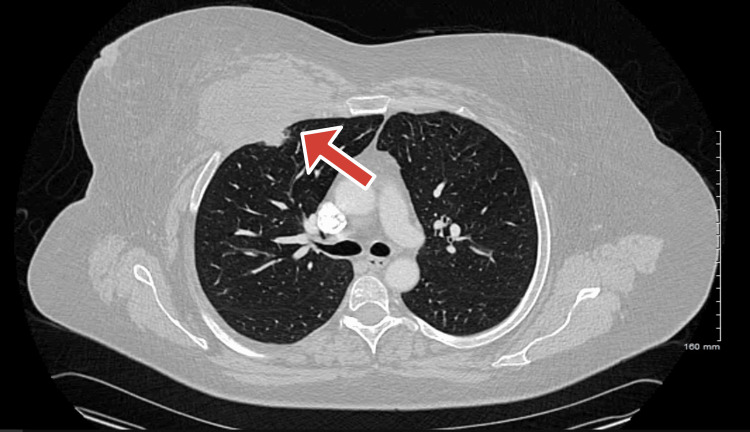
Chest CT with lobulated mass eroding the T3 rib and indenting the pleura, with extension between T3 and T6 ribs and an associated 3.3 × 2.1 cm anterior mediastinal lesion Arrow pointing at lobulated mass CT, computed tomography
